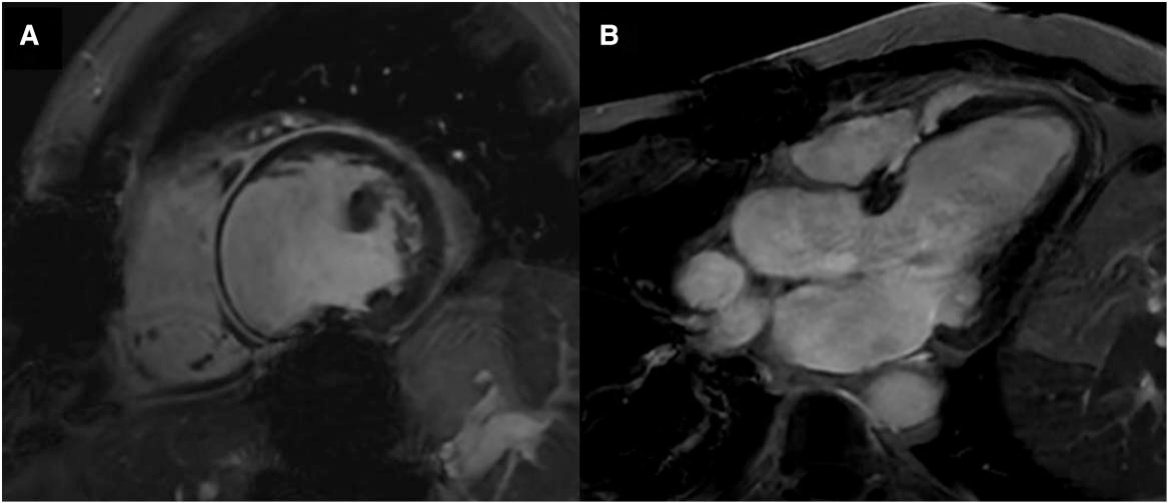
(*A* and *B*) Cardiac magnetic resonance imaging highlighting interval progression of an extensive predominantly subepicardial late gadolinium enhancement involving the basal to mid anterior, anteroseptal, and septal walls.
